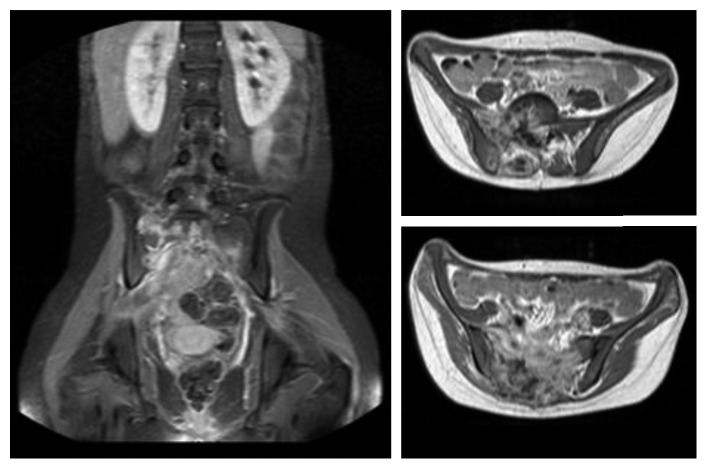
Selection of characteristic coronal and axial T1-weighted MRI slices of the Ewing Sarcoma.
